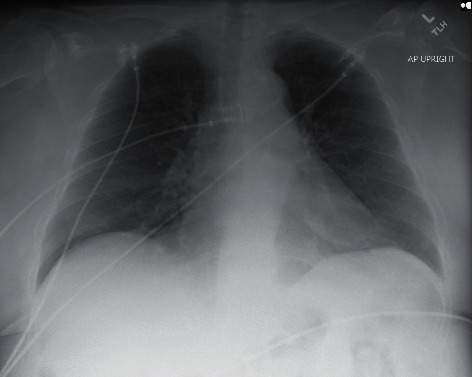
Chest X-ray of our patient showing diffuse nodular involvement of the lungs.
